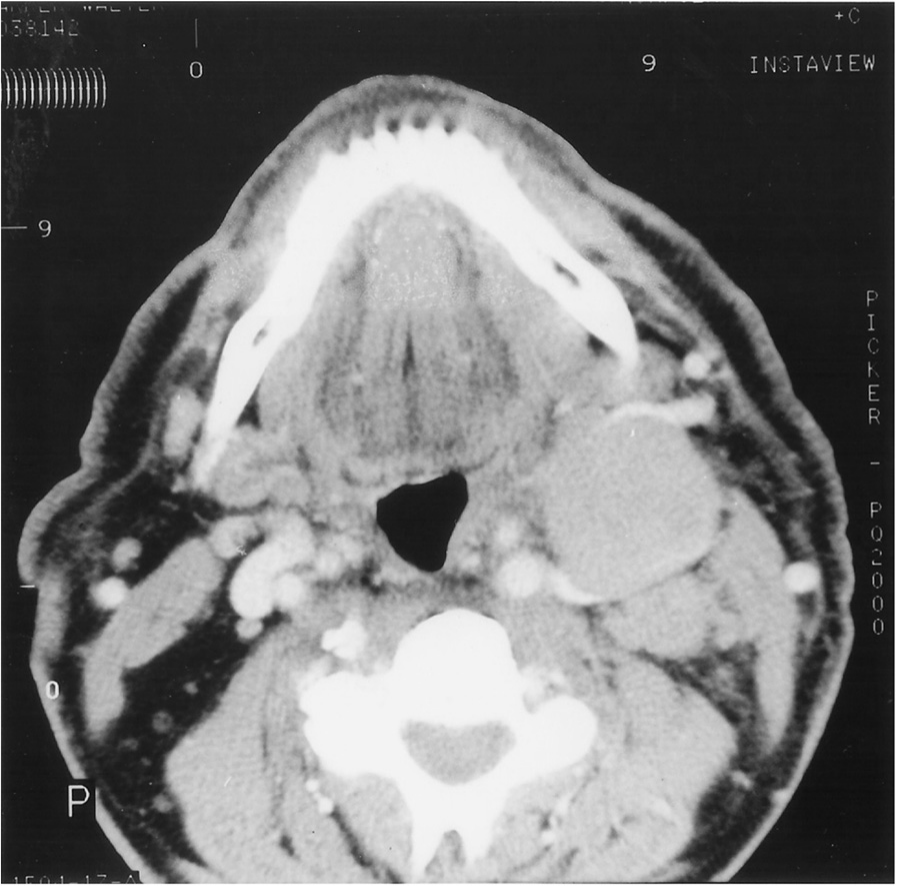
Computed tomography scan showing a well circumscribed lymph node in the left side of the neck.
